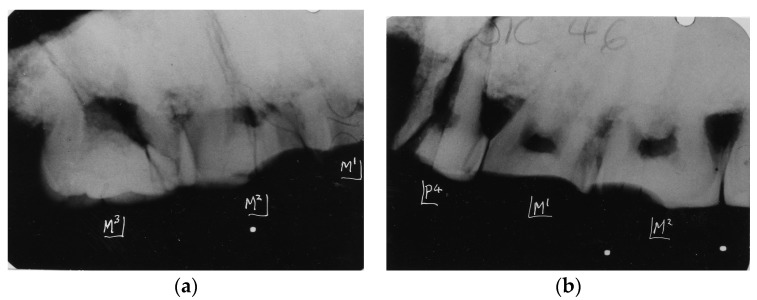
(a) Paranthropus robustus. Kromdraai. SK 46. Radiograph of right maxillary teeth revealing graded wear from M1 (severe) to minor wear on M3; (b) Paranthropus robustus. Kromdraai. SK 46. Radiograph of left maxillary teeth. An unusual pattern of severe wear confined to the mesial half of M2 is seen that requires some explanation. The less worn distal half of this tooth and M3 suggests absence of the corresponding occluding mandibular M2 and M3. Thereby, unusual wear patterns can reveal peculiar phenomena of abrasive wear.
